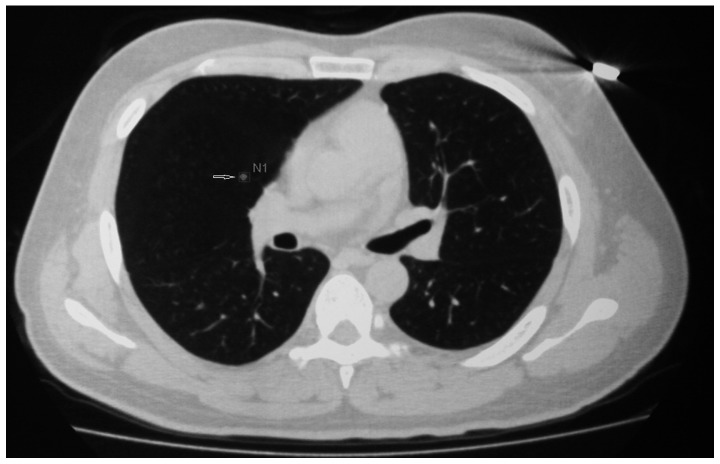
Case one. Chest computed tomography scan performed at a follow-up visit 21 months after the first surgery showing numerous metastatic nodules in diffuse and random distributions in each lung.
